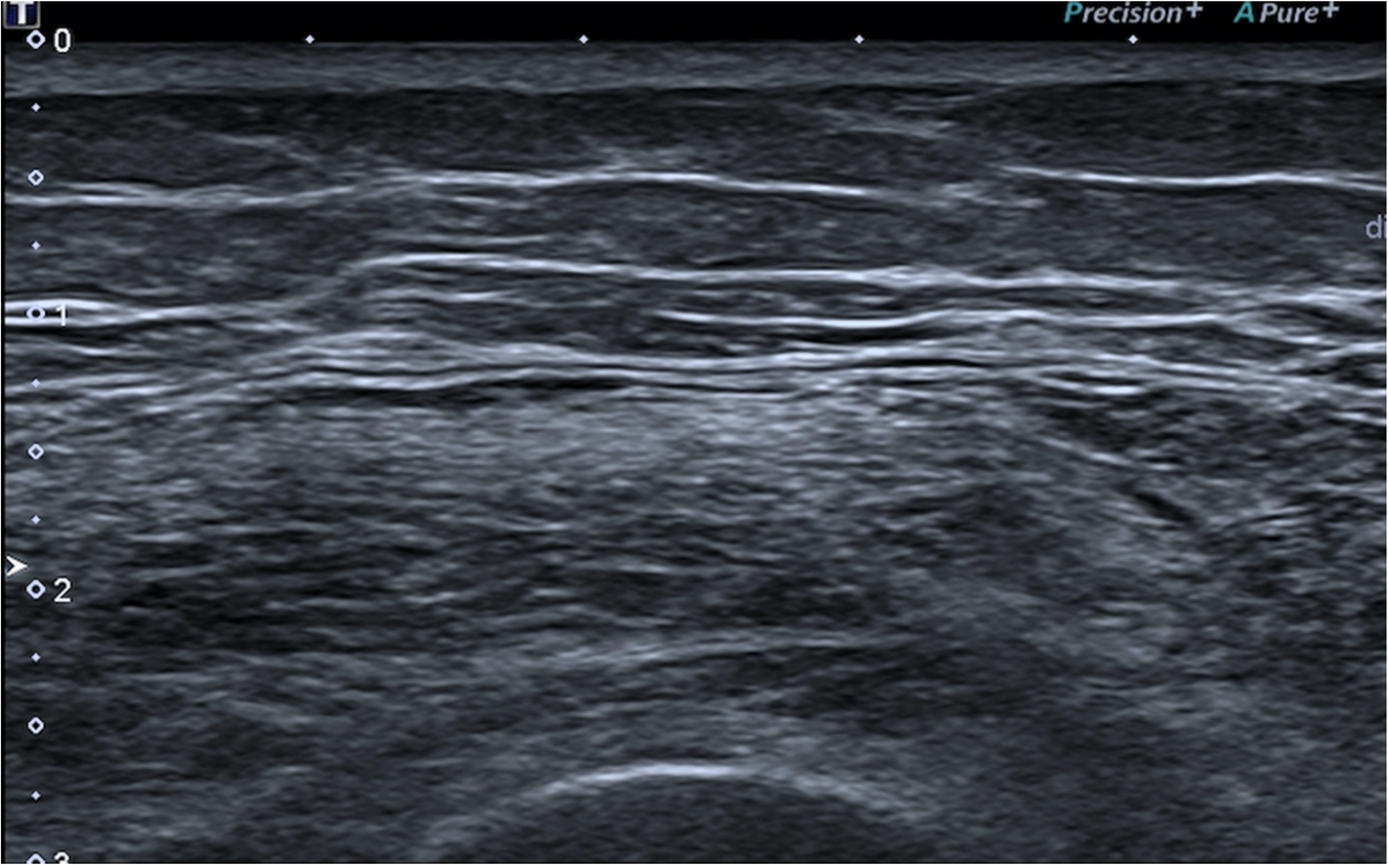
B mode Ultrasound showing a transverse view of the Vastus Lateralis in a patient with Inclusion Body Myopathy. Muscle echogenicity is increased (appears brighter) and there is loss of muscle bulk
